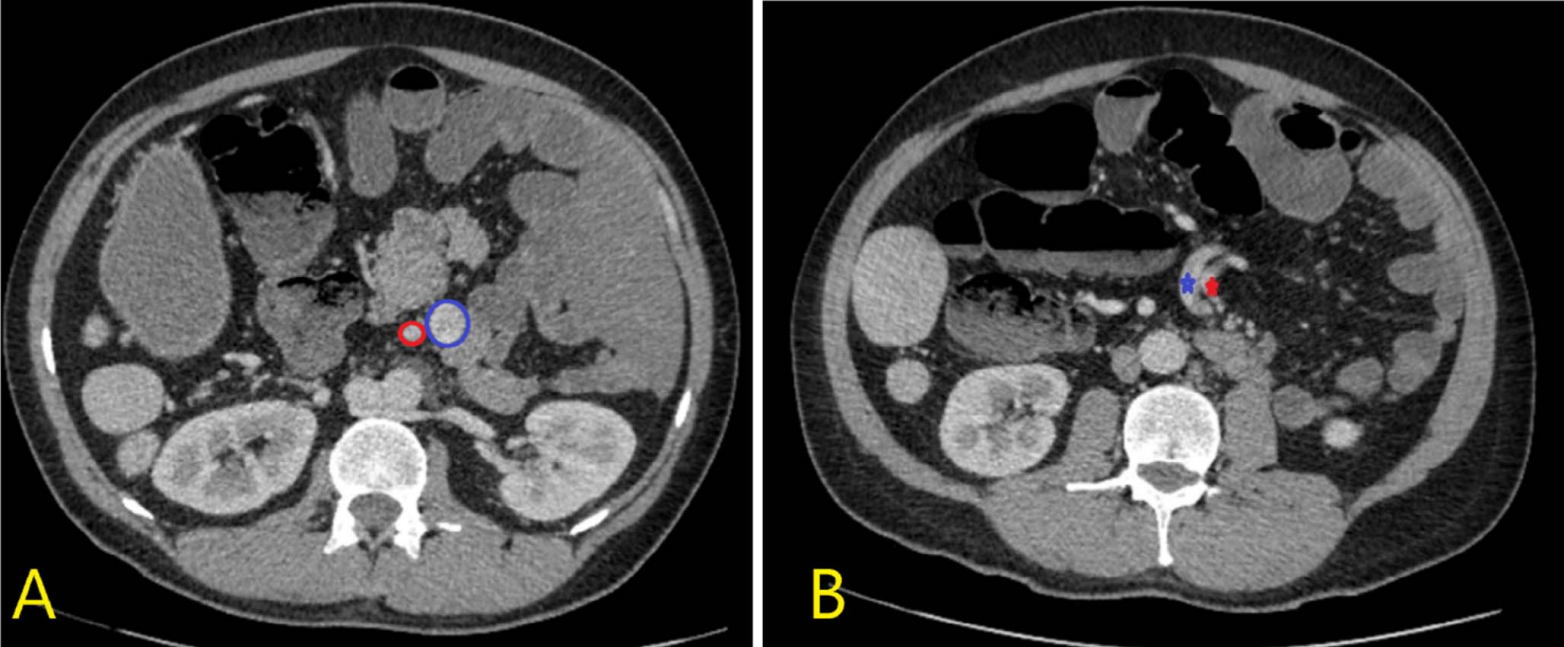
(A) Fat-saturated contrast-enhanced computed tomography image shows the abnormal relationship between the superior mesenteric artery (red circle) and the superior mesenteric vein (blue circle). (B) In a successive section in the same phase, the superior mesenteric vein (blue star) rotates around the superior mesenteric artery (red star) from posterior to anterior.
